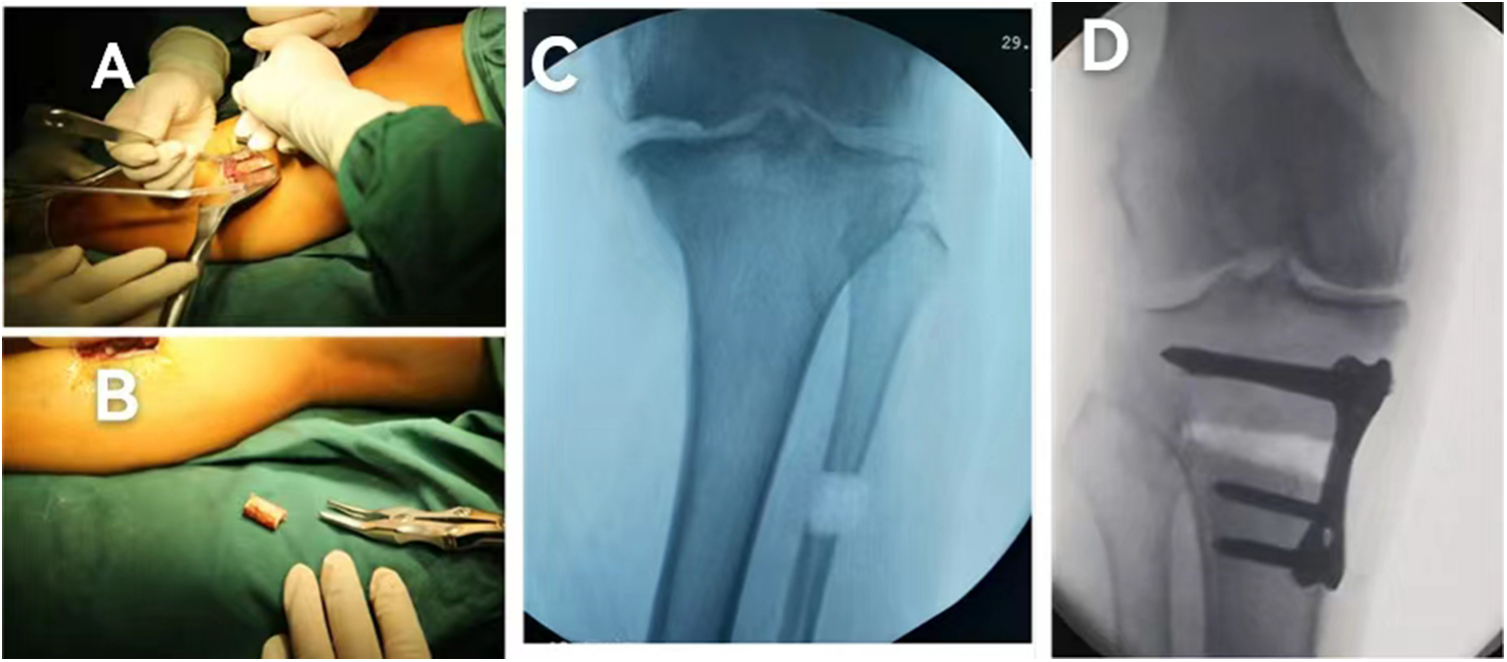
Open-wedge high tibial osteotomy and proximal fibular osteotomy. (A) Exposure of the fibula, (B) the 1.5-cm fibula is cut off with a pendulum saw, (C) the intraoperative radiograph of proximal fibular osteotomy, and (D) the osteotomy is fixed with a four-point support plate.
